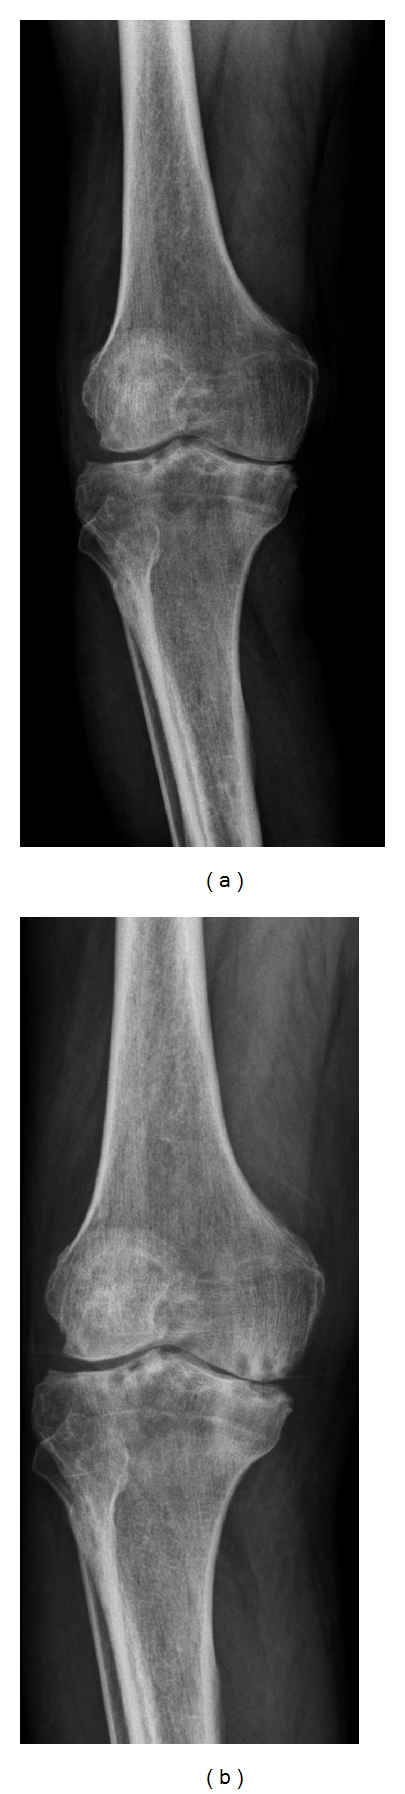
(a) Standing knee radiograph showed multiple patched subchondral lesions of the distal femur and proximal tibia, joint space narrowing, and definite osteophyte. (b) After disappearing of the infection sign, standing knee radiograph showed aggravation of intra-articular deformity.
